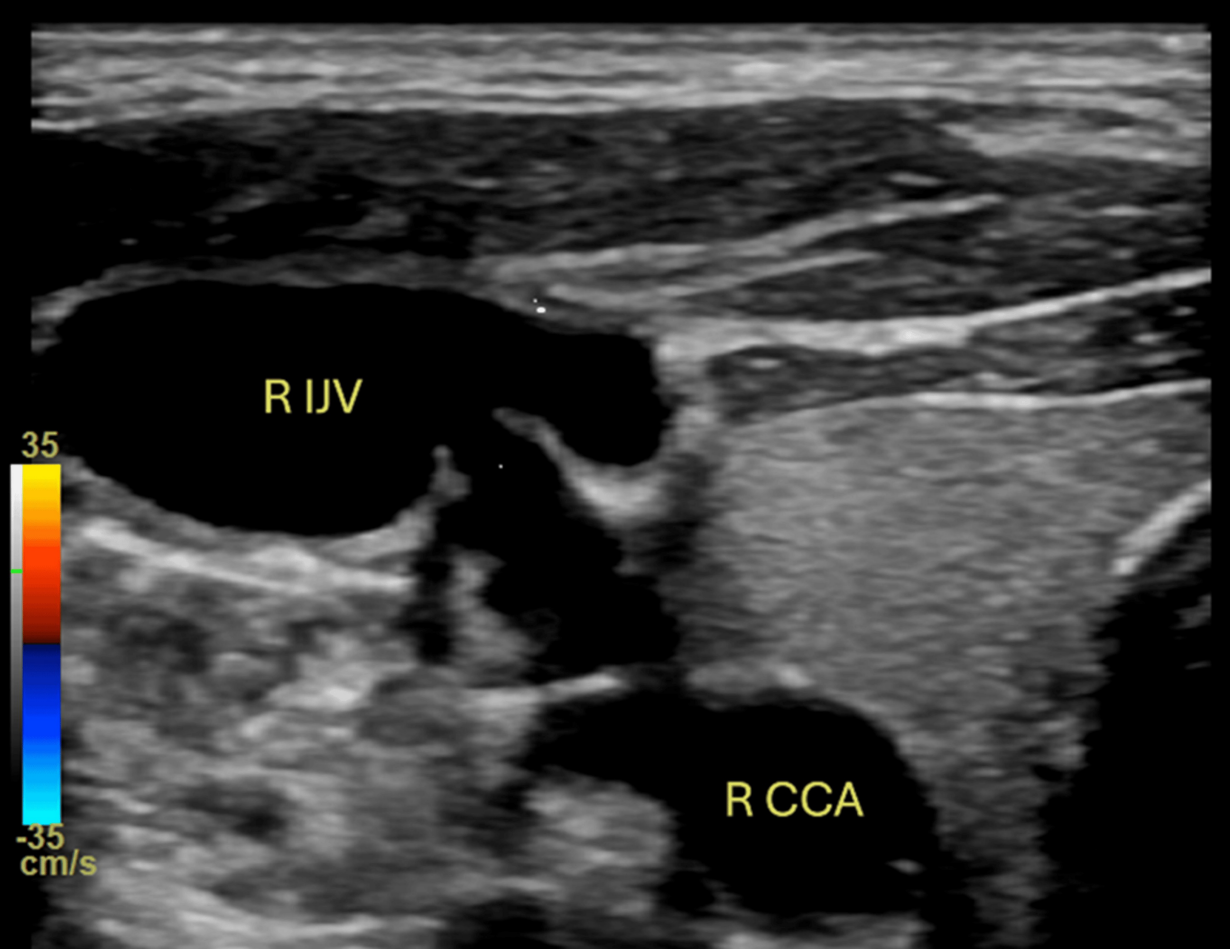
Carotid duplex ultrasound (grey scale) R IJV: right internal jugular vein; R CCA: right common carotid artery
